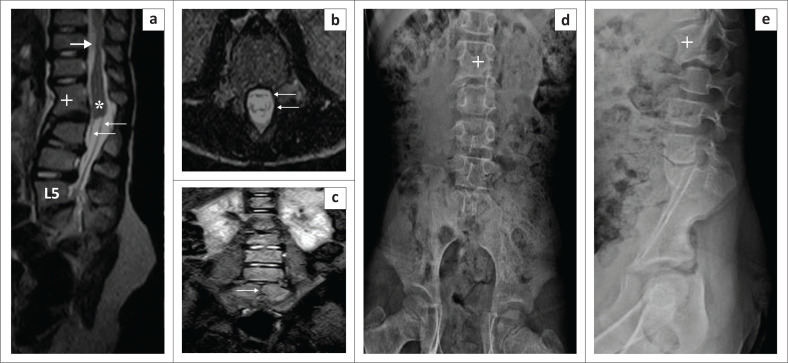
Type I caudal regression syndrome. Sagittal T2-weighted image (T2WI) (a) and radiographs (d, e) show non-visualisation of the sacrum (beyond S1) and coccyx with a L1–L2 block vertebra (+). There is mild anterior wedging of the L3 vertebra. Coronal short tau inversion recovery (STIR) (c) shows a vertical midline cleft (thin black arrow) in the S1 vertebral body. Sagittal T2WI (a) shows attenuation of the spinal cord (solid white arrow) from the T10 to T12 vertebral levels and a thickened conus medullaris (*) with abrupt termination at the lower end of the block L1–L2 vertebra. The nerve roots follow an atypical course having a ‘double-bundle shape’ (a, b) (thin white arrows).
